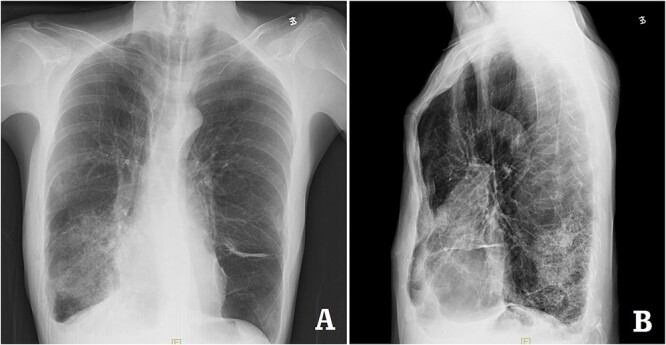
X-ray examination after the bars were taken out. (A) Posteroanterior radiograph; and (B) lateral radiograph.
